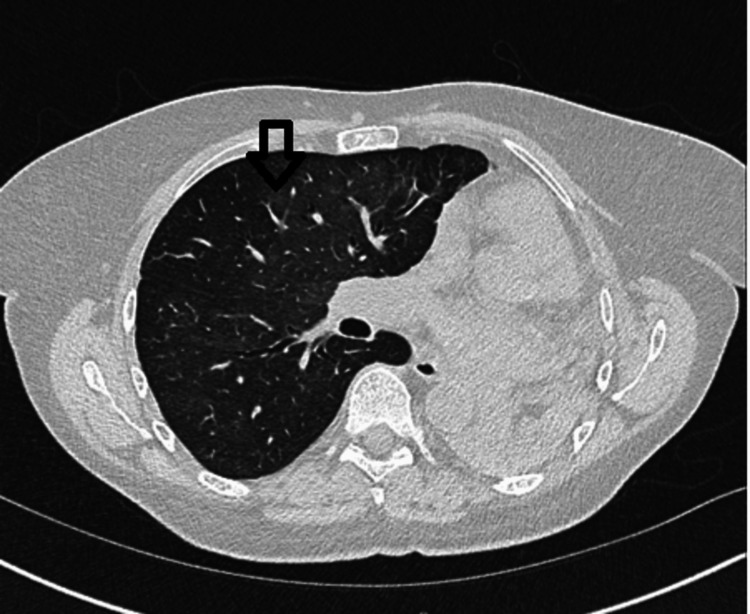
High-resolution computed tomography performed two weeks after initiation of high-dose steroids and rituximab, showing marked improvement in ground-glass changes in the right lung (arrow).
